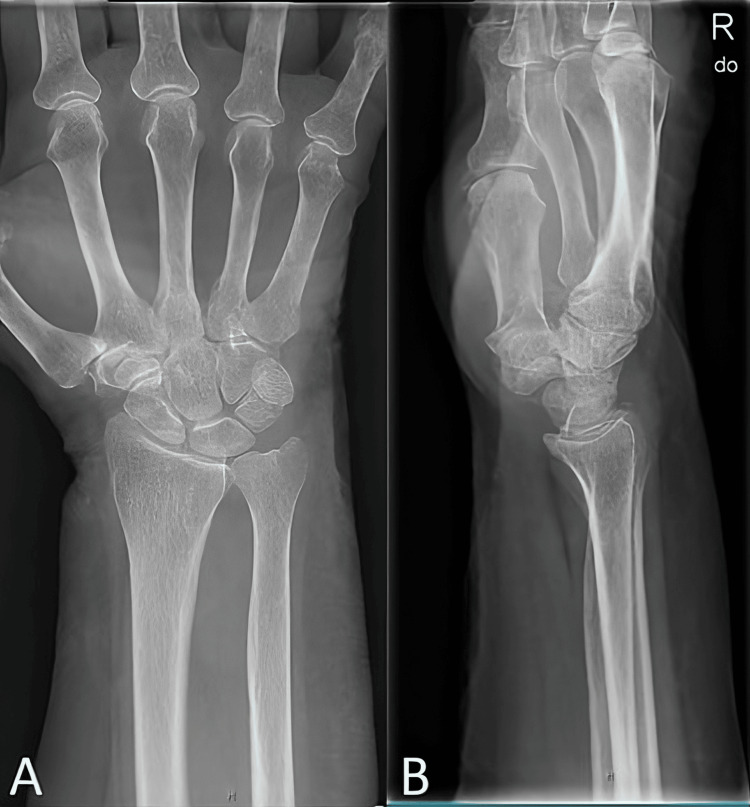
Posteroanterior (A) and lateral (B) radiographs of the right wrist, showing the proper anatomical position of the DRUJ at three weeks after closed reduction. DRUJ: distal radioulnar joint
